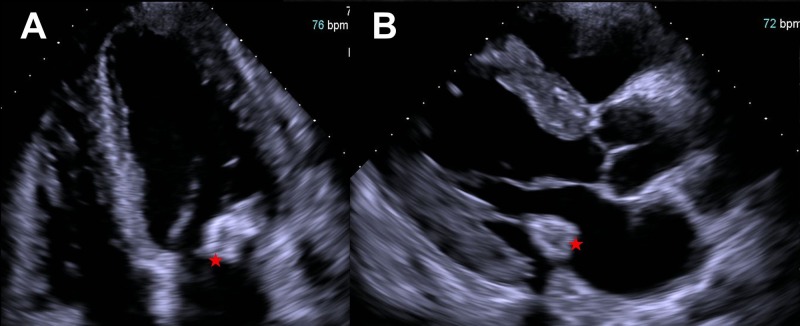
Two-dimensional transthoracic echocardiography. Two-dimensional transthoracic echocardiography - apical four chamber (A) view and long-axis parasternal (B) view demonstrated a nonmobile calcified mass (2.45 cm x 1.60 cm) located over the posterior mitral annulus, with central areas of echolucency (red asterisks) extending into the mitral inflow area.
